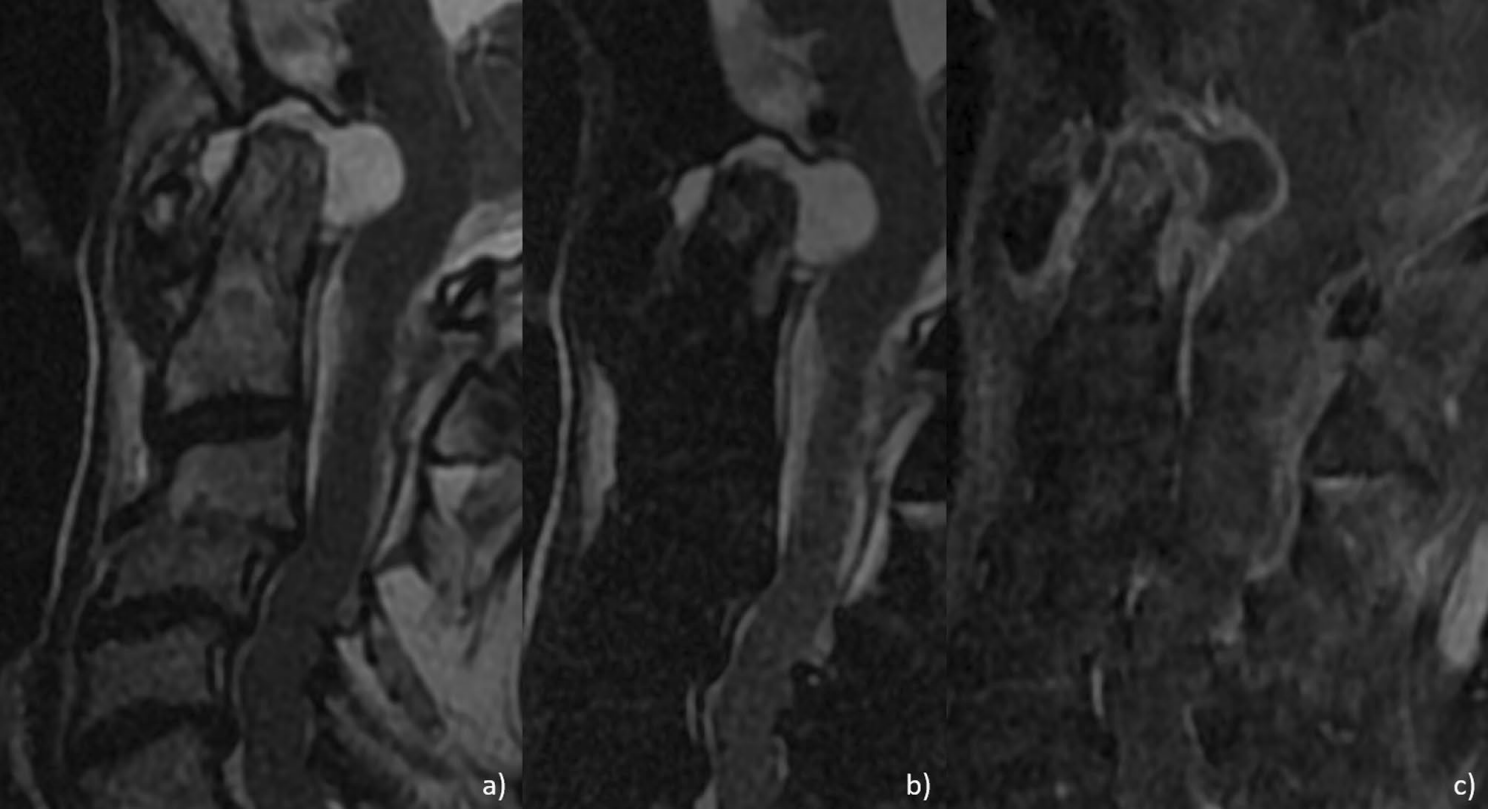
Sagittal T2 (a), fat-saturated T2 (b) and contrast enhanced T1 (c) MRI images showing marked synovial hypertrophy and effusion, with edema and erosions of the odontoid peg.
